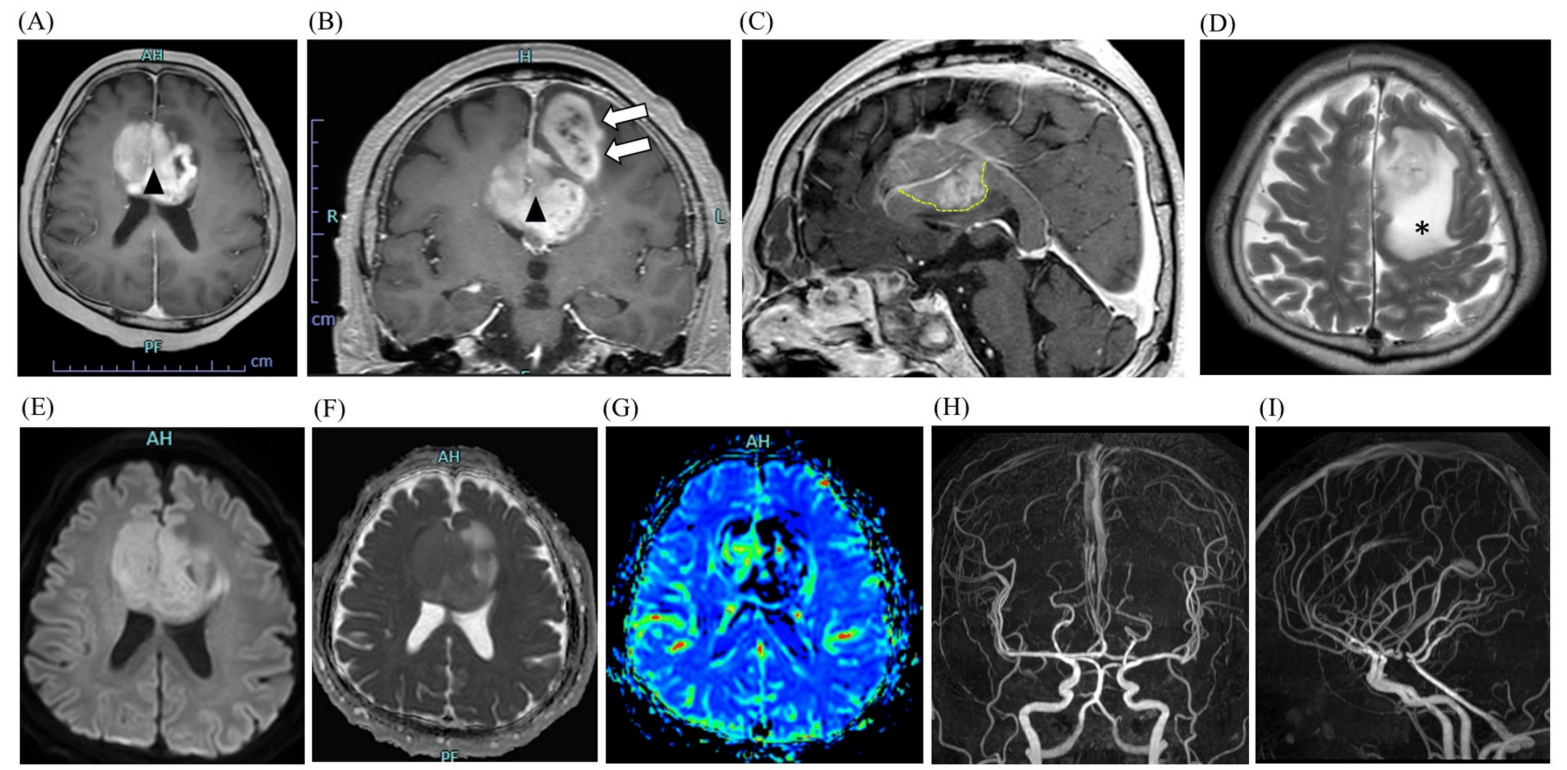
Preoperative MRI findings. Initial brain MRI demonstrates well-enhanced mass lesions (maximum diameter: 65 mm) involving the bilateral anterior cingulate gyri (arrowheads) and the left superior frontal gyrus (blank arrows) on post-contrast T1-weighted axial (A), coronal (B), and sagittal (C) images. Notably, the tumor extends into the anterior body of the corpus callosum (C, dotted line), with surrounding edema visible on the T2-weighted image (D, asterisk). Additional sequences reveal mild diffusion restriction on the diffusion-weighted image (E) and the apparent diffusion coefficient map (F), along with mildly elevated cerebral blood volume as assessed by DSC-MRI (G). TOF-MRA frontal (H) and lateral (I) views show no apparent abnormalities in the main arteries. DSC: Dynamic susceptibility contrast; MRA: Magnetic resonance angiography; TOF: Time-of-flight.
